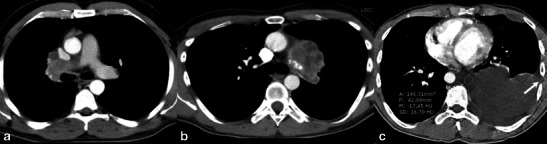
CT images of primary intrathoracic sarcomas. A 41-year-old man (a) with pulmonary artery undifferentiated sarcoma, lesion showing irregular shape and necrosis, with component that invades the lumen of the right pulmonary artery (intraluminal tumour). A 38-year-old man (b) with a mediastinal osteosarcoma, with irregular shape, lobulated margins, necrosis and intratumoural calcifications in the lesion’s medial aspect. A 26-year-old man (c) with liposarcoma, presenting a lesion of undetermined shape and undefined margins, infiltration of the chest wall (*arrow*) and components of low attenuation (ROI)
